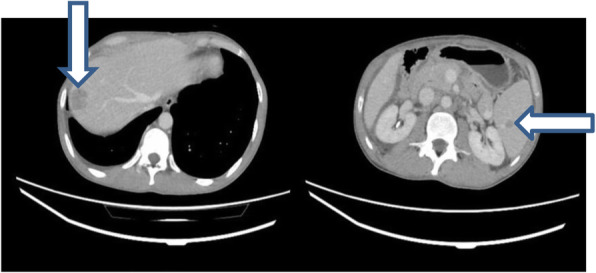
Spiral CT scan showed well-defined solid lesions in the liver (vertical arrow) and spleen (horizontal arrow)
